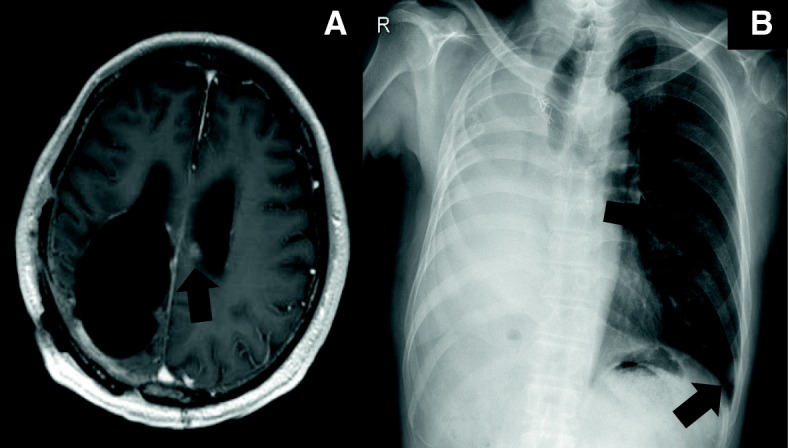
a Brain magnetic resonance imaging (MRI) shows meningeal dissemination in left lateral ventricle (arrow). b Chest x-ray scan shows two nodule shadows in left lower lung field (arrow)
